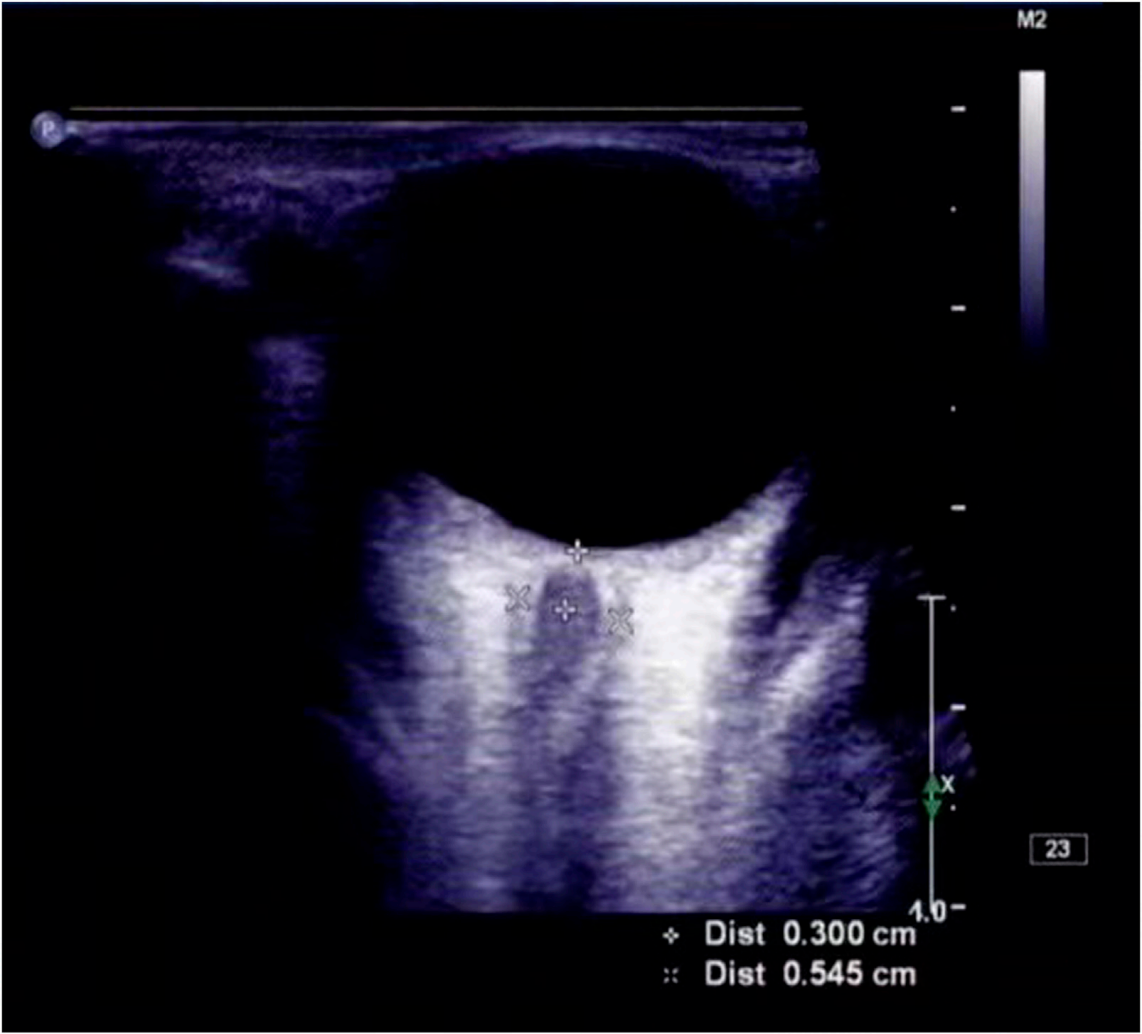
ONSD was measured by ultrasound. The ONSD, as measured with transorbital ultrasonography, was 0.545 cm, in this patient (a man in his early 60s) with acute ischemic stroke.
